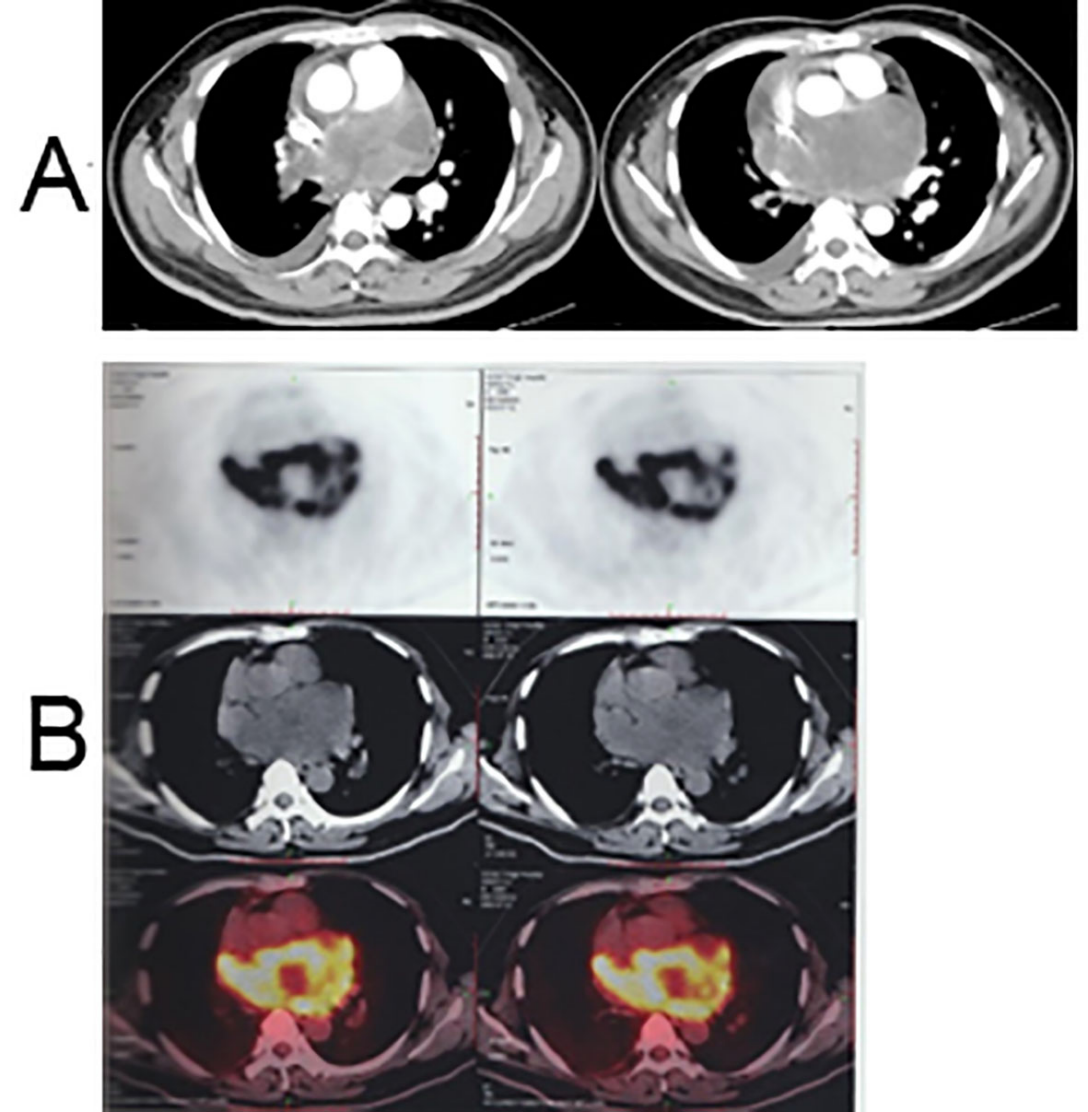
Chest CT and PET-CT scan before treatment. (A) Enhanced chest CT scan images: a posterior mediastinal tumor measuring 11.9 × 7.6 cm with involvement of the right pulmonary arteries, accompanied by pericardial effusion and minor pleural effusion on both sides; (B) PET-CT scan image: a large soft tissue mass in the posterior mediastinum with increased metabolism (SUV_max_: 26.3), involving the right pulmonary artery trunk and associated arteries.
